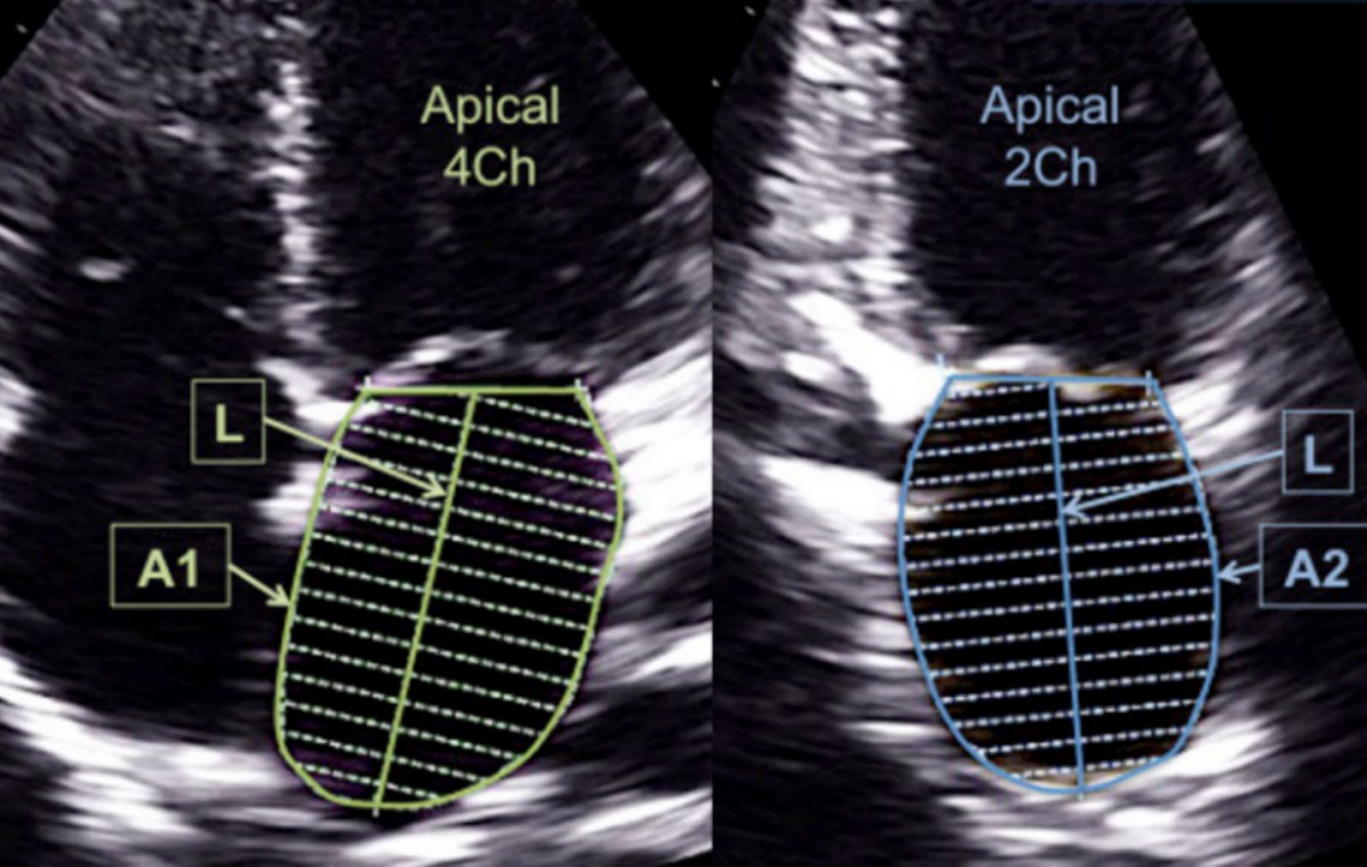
2D ECHO assessment of LA maximal volume by tracing of left atrium endocardium in apical four-chamber and two-chamber view
